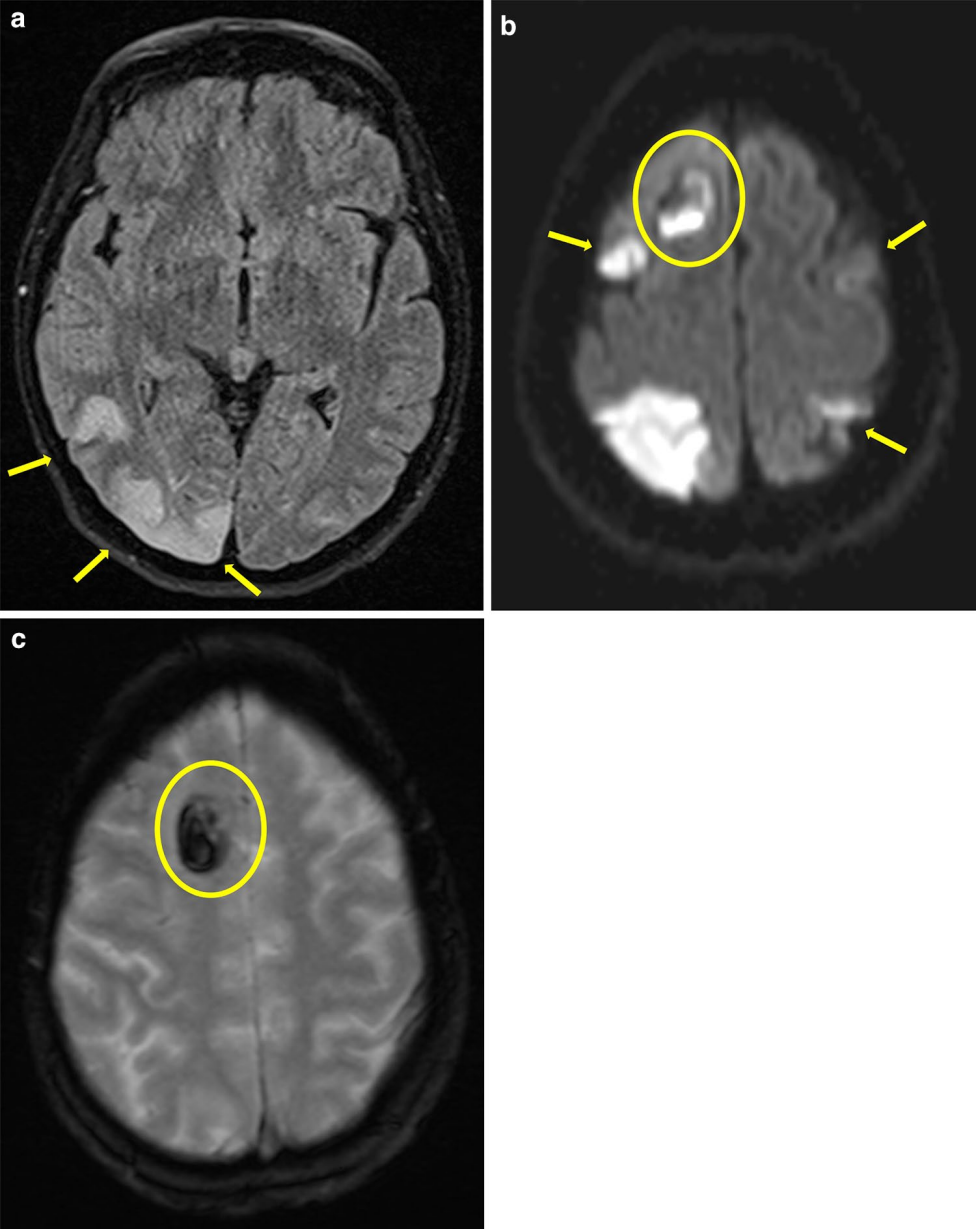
The following magnetic resonance imaging (MRI) findings were obtained in a 55-year-old female patient with reversible cerebral vasoconstriction syndrome (RCVS). They reflect the most common complications of RCVS. a Occipital, predominantly right-lateralized fluid-attenuated inversion recovery (FLAIR) hyperintensities (transverse; yellow arrows), consistent with posterior reversible encephalopathy syndrome (PRES). b Hyperintensities on diffusion-weighted imaging (DWI) and FLAIR (hypointense on ADC (apparent diffusion coefficient) mapping) in the bilateral anterior circulation area (middle cerebral artery), resembling toxic edema (DWI, transverse; yellow arrows). c An atypically located intracerebral hemorrhage in the right frontal lobe on T2*-weighted MRI (transverse), also hyperintense on DWI (yellow ellipse in b, c)
